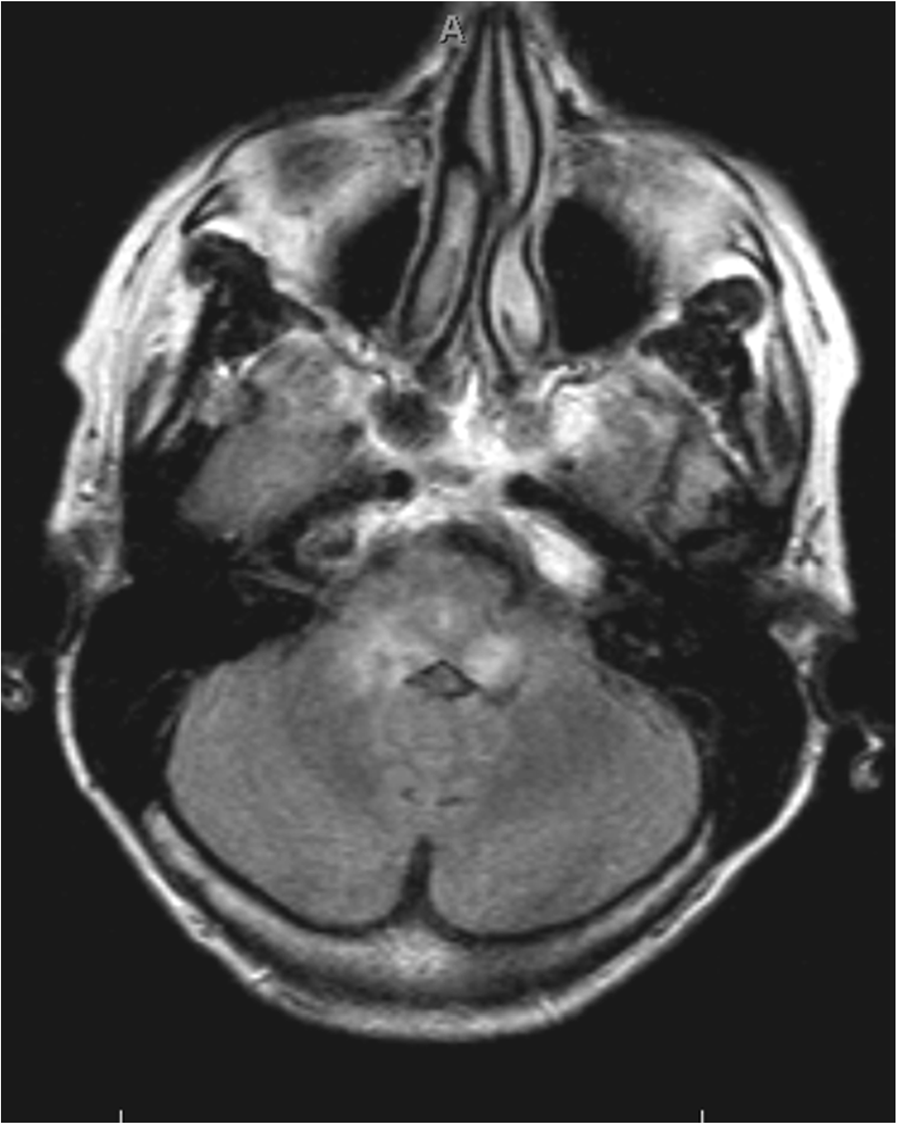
MRI cerebrum Fluid Attenuated Inversion Recovery (FLAIR), diffuse white matter lesions with extension into the pons and medulla oblongata
